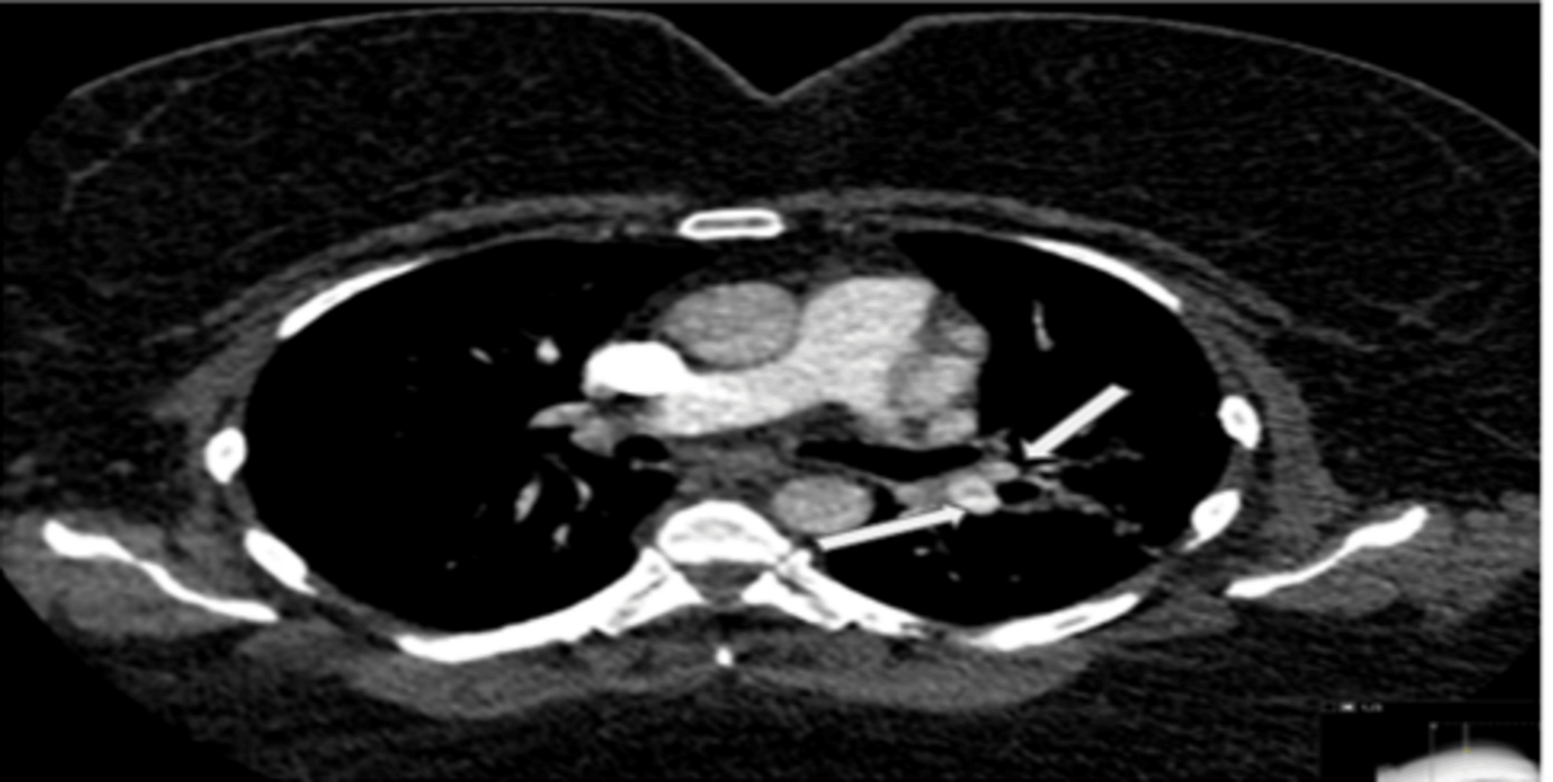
Axial CT pulmonary angiogram showing partial filling defects within the left lower lobe and lingular segment branches
